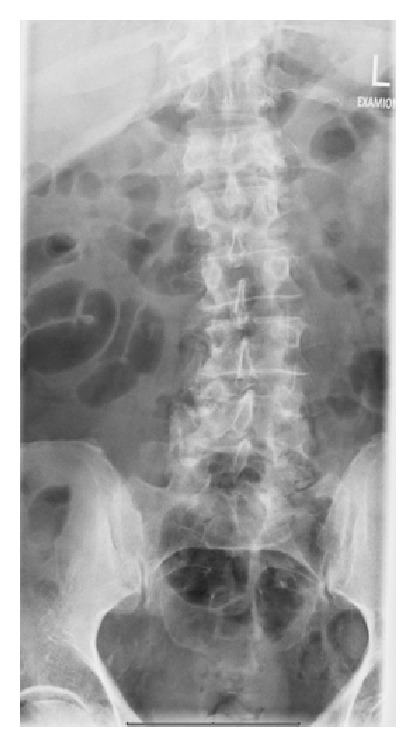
Lumbar spine radiographs on admission showed degenerative changes.
